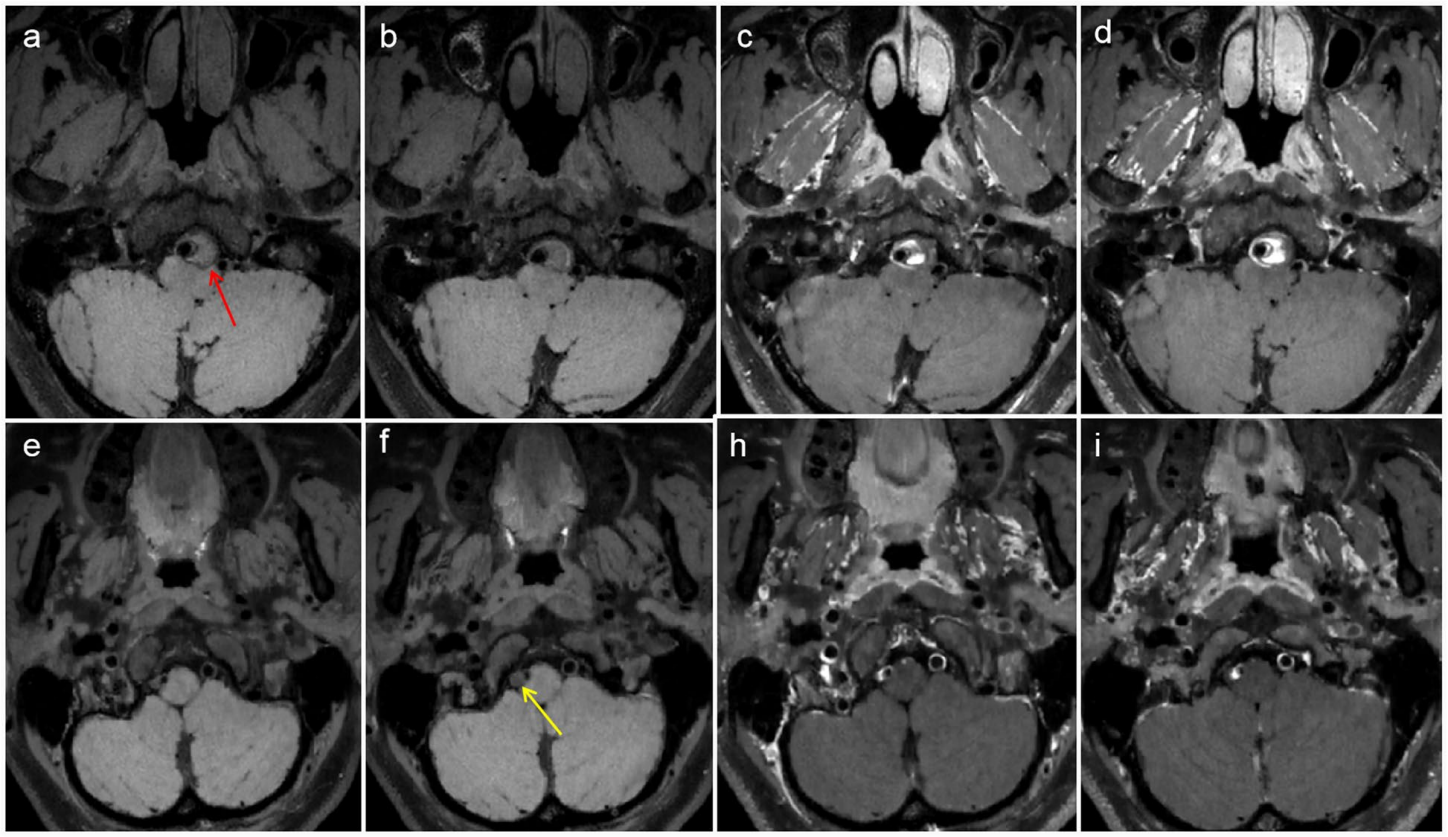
HR-MRI images of two patients. a-d HRMR-VWI taken at admission of a 55-year-old male patient who presented with repeated headache for 3 years. A thrombotic aneurysm of the V4 segment of the right vertebral artery, compressing the brain stem (mass effect) was observed. e-i HR-MRI taken during physical examination of a 43-year-old female patient with a history of hypertension showed a thrombotic aneurysm of the V4 segment of the right vertebral artery, and the compressed vessel was slightly narrowed
